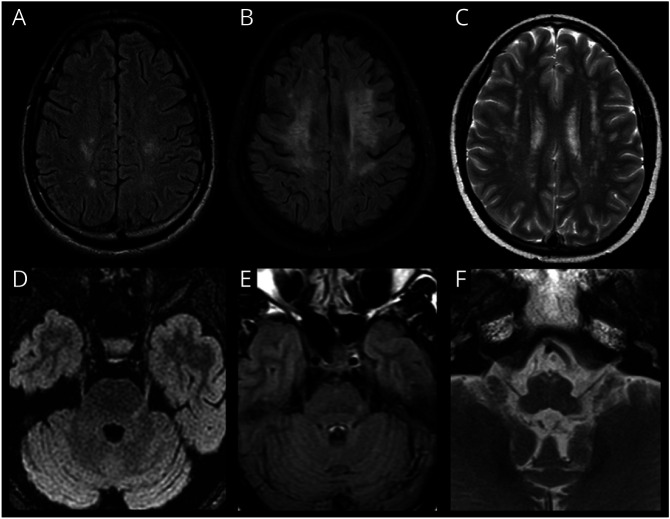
MR Imaging in HTLV-1–Related White Matter Disease Axial FLAIR (A and B) images of two different patients with HTLV-1 demonstrated symmetrical lesions in the precentral white matter with the second patient (B) demonstrating more extensive confluent lesions in the frontal white matter. Axial T2w image (C) of a 24-year-old woman with chronic HAM since 12 years of age demonstrates multiple partially confluent high T2w lesions in the deep and subcortical frontoparietal white matter. Axial FLAIR images of two different patients with HAM (D and E) both demonstrating lesions along the left trigeminal root entry zone. Axial T2w image of a patient with HAM and encephalopathy (F) demonstrating symmetrical lesions in the medullary pyramids along the path of the corticospinal tracts.
